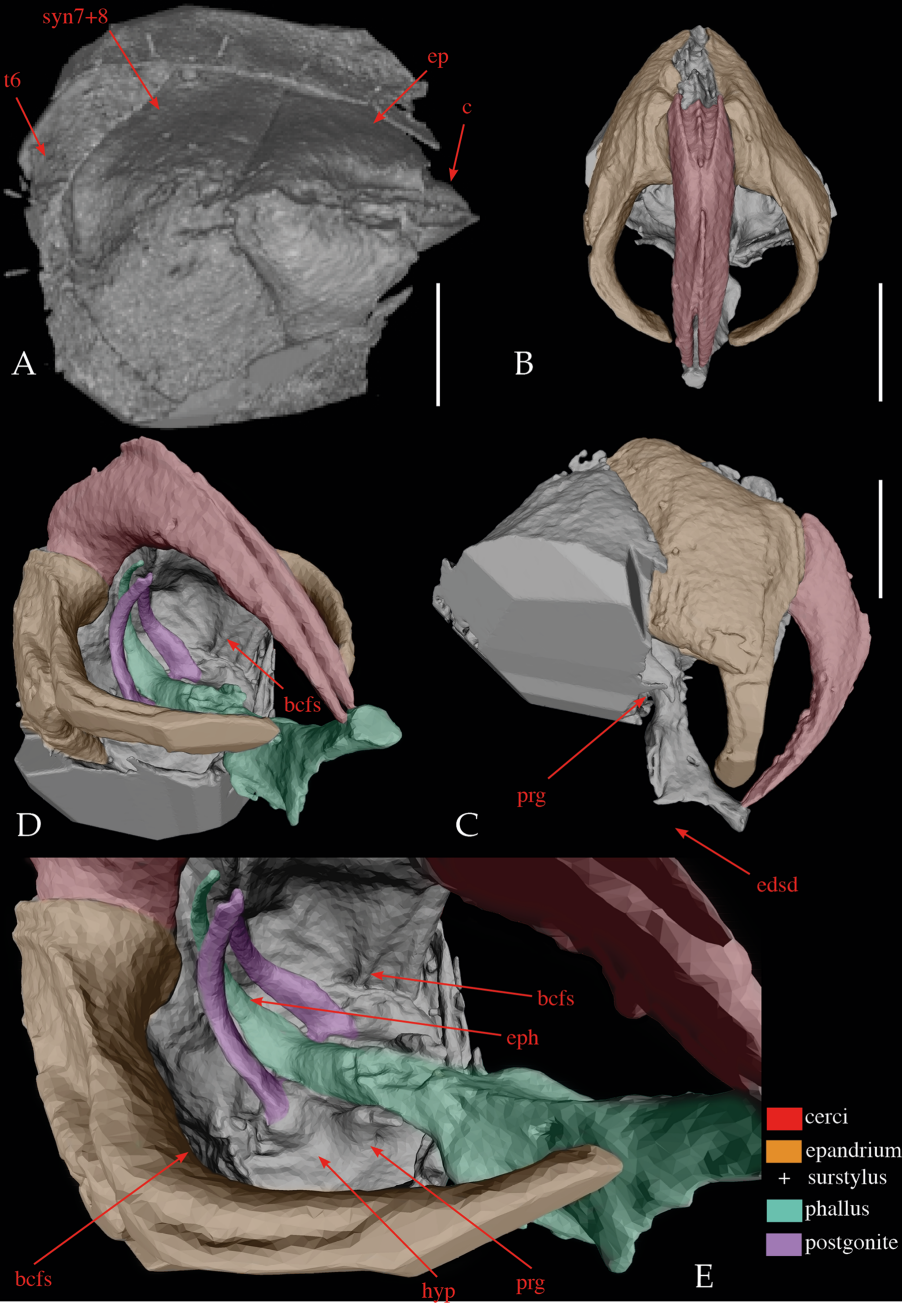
Holotype of *Mesembrinella caenozoica* sp. nov., CT scan of the terminalia. (A) tergite 6, sytergosternite 7+8, epandrium and cerci in posterior view. (B) epandrial complex in posterior view. (C) epandrial complex and phallus in left lateral view. (D) terminalia in left ventrolateral view. (E) detail of phallus and hypandrial complex in left ventrolateral view. Abbreviations: bcfs = bacilliform sclerite; c = cerci; edsd = tip of extension of dorsal sclerite of distiphallus; ep = epandrium; eph = epiphallus; hyp = hypandrium; prg = pregonite; syn7+8 = syntergosternite 7+8; t6 = tergite 6. Scale bars: 0.4 mm.
